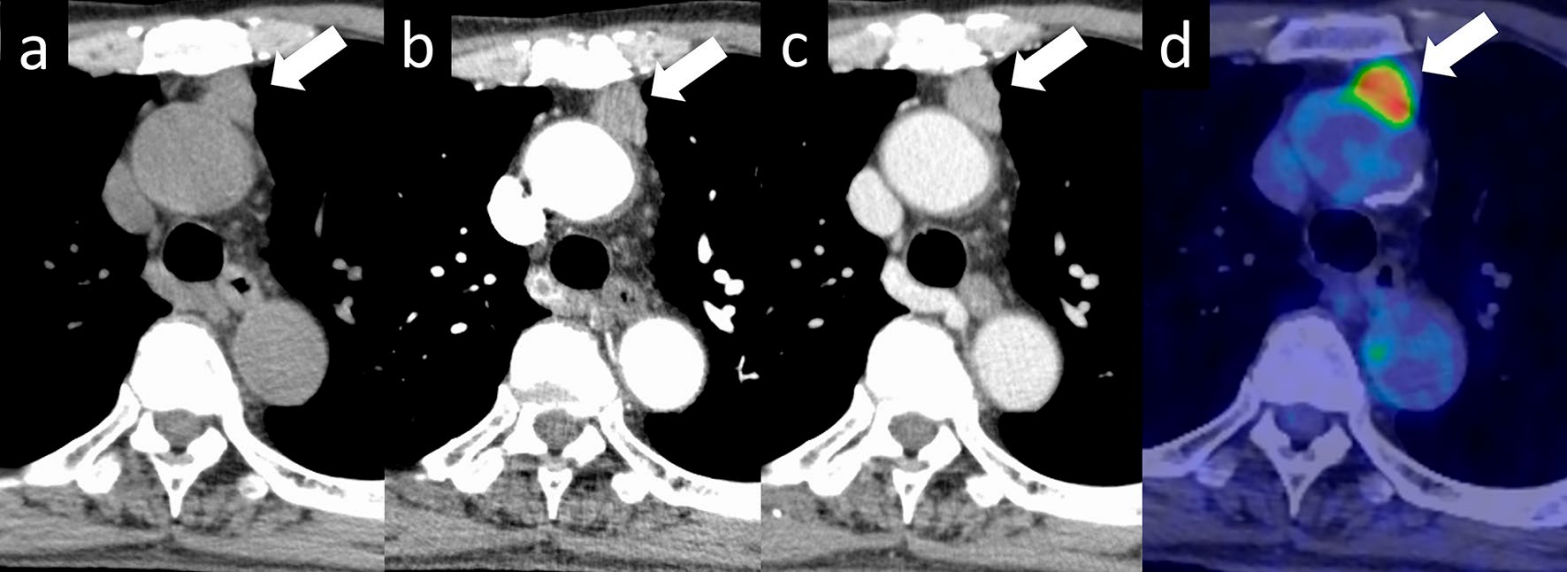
Thymoma (type B3). A 71-year-old man with type B3 thymoma. a–c On contrast-enhanced dynamic CT, a 2-cm, lobulated nodule with homogenous attenuation shows gradual enhancement (arrow). The internal density of the nodule is homogeneous. Contrast-enhanced dynamic CT of the nodule shows gradual enhancement. d 18F-FDG PET shows high FDG uptake in the perivascular nodule (SUVmax = 9.74) (arrow). CT, computed tomography; 18F-FDG PET, 18F-fluorodeoxyglucose positron emission tomography; SUVmax, maximum standardized uptake value
